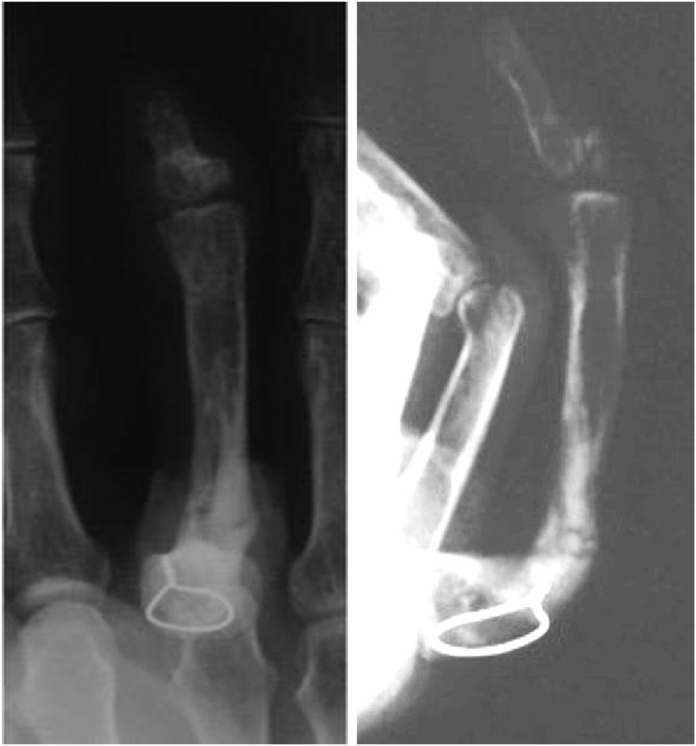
X-ray after bone union, the right image shows the lengthened proximal phalanx bent at an angle of 80°.
